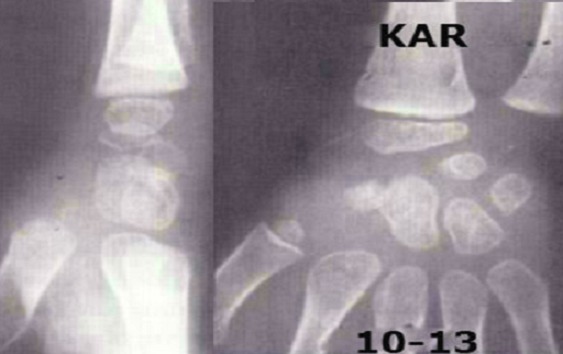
Aspect radiographique à 10 mois de recul, le pole supérieur du scaphoïde n'est pas visible, l'espace radio/grand os reste diminué
